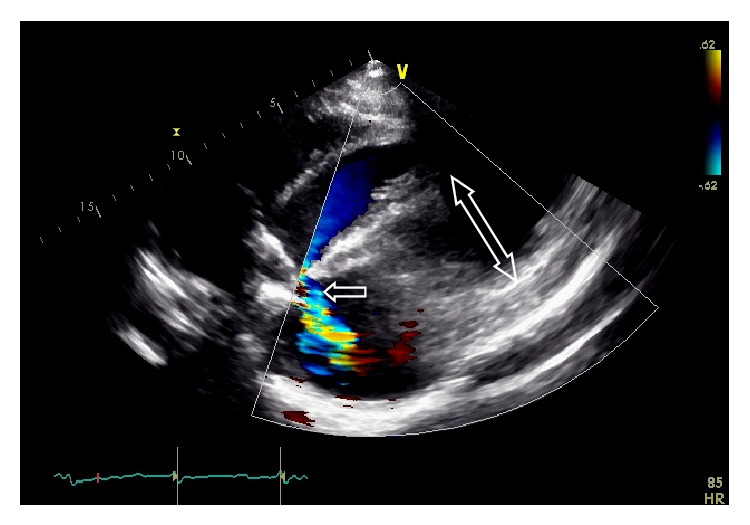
Color Doppler image shows flow from the left ventricle through the fistulous tract (single head arrow) into the pericardial space.
